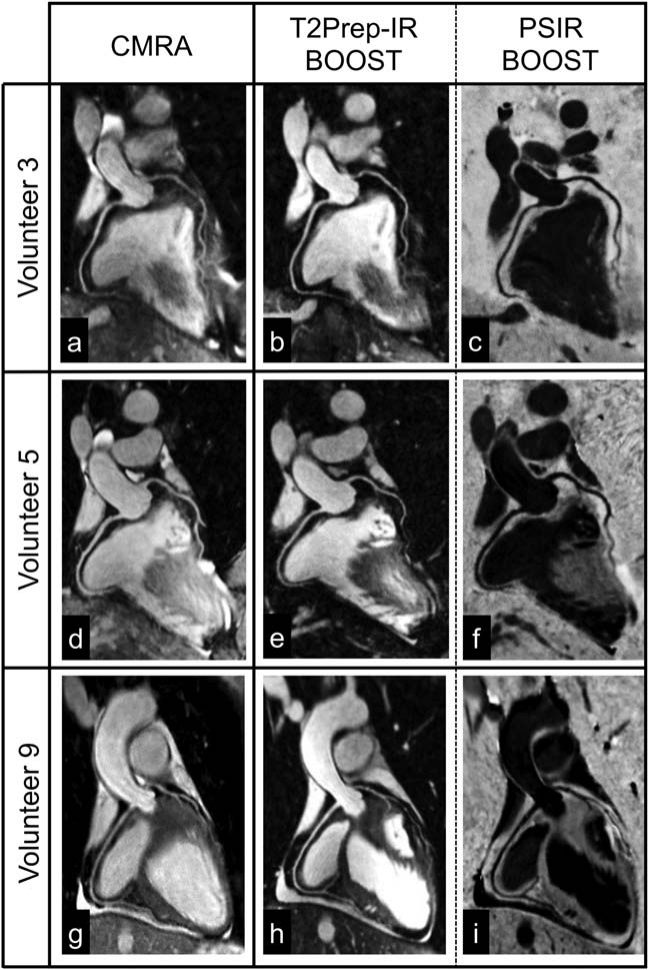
Reformatted coronary depiction in three representative healthy volunteers obtained with a conventional T_2_‐prepared bright‐blood CMRA acquisition (a, d, g) and the proposed BOOST sequence for simultaneous bright‐blood (T_2_Prep‐IR BOOST datasets in b, e, h) and black‐blood (PSIR BOOST datasets in c, f, i) whole‐heart MRI. Quantified CNR_blood‐myo_ significantly improved with the proposed T_2_Prep‐IR BOOST approach in comparison to the conventional CMRA, thus leading to a higher quantified coronary percentage vessel sharpness (%VS) for both right and left coronary arteries. In the PSIR BOOST images in (c, f, i), the efficacy of blood signal suppression can be appreciated along multiple portions of the coronary tree. BOOST, Bright‐blood and black‐blOOd phase SensiTive; CMRA, conventional coronary MR angiography; IR, inversion recovery; myo, myo, myocardium; PSIR, phase‐sensitive inversion recovery; T_2_Prep, T_2_ prepared.
